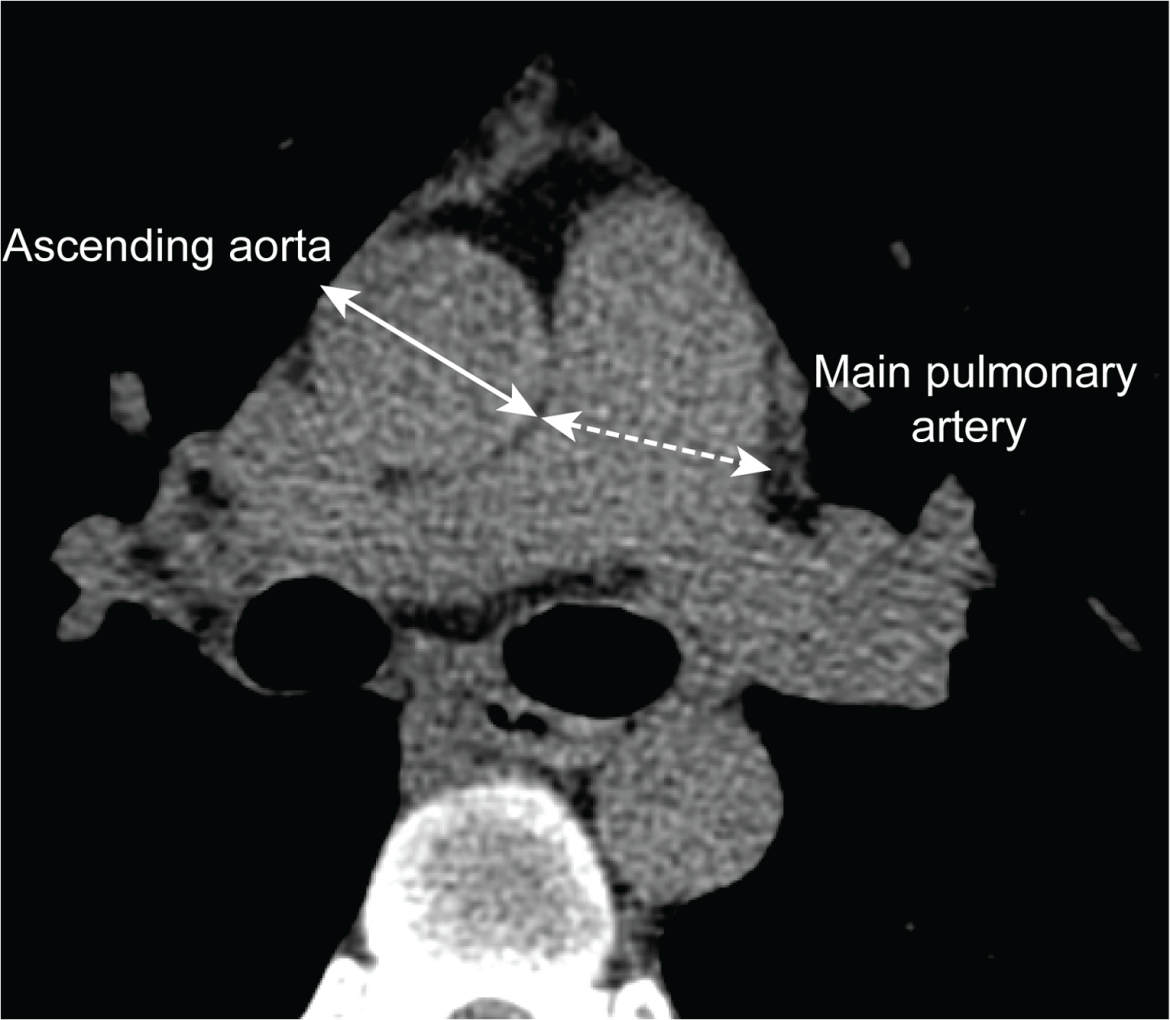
Measurement of the pulmonary artery and ascending aorta diameters. Pulmonary artery and ascending aorta diameters were assessed on a transverse CT image at the level of the pulmonary artery bifurcation. Vessel diameters were obtained by measuring the widest diameter vertical to the long axis of the main pulmonary artery, using a computer caliper. mPA, main pulmonary artery; Ao, aorta
